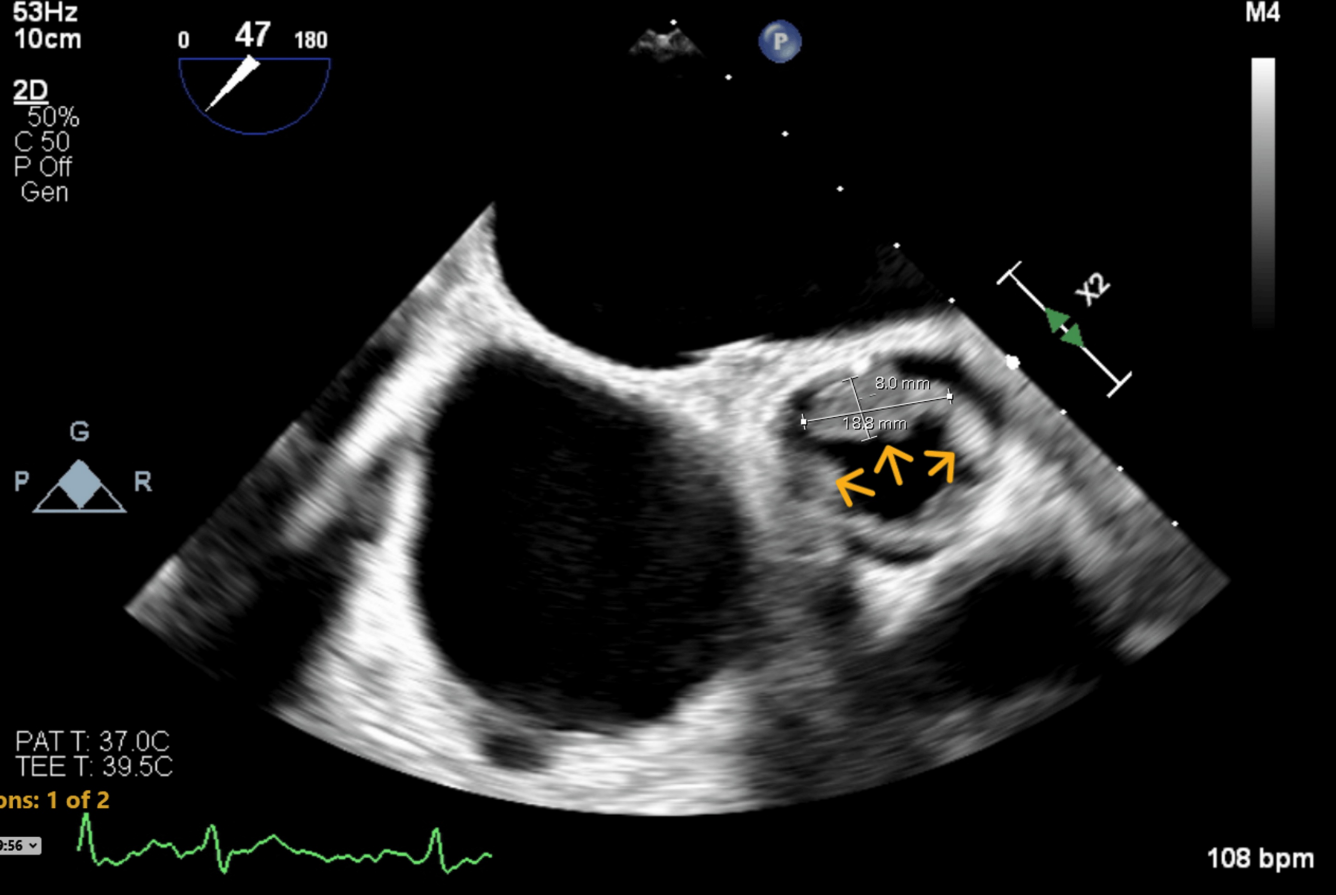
Transesophageal echocardiogram (aortic valve short axis view) Arrows indicate the aortic valve leaflet vegetation (center) and leaflet thickening (sides).
